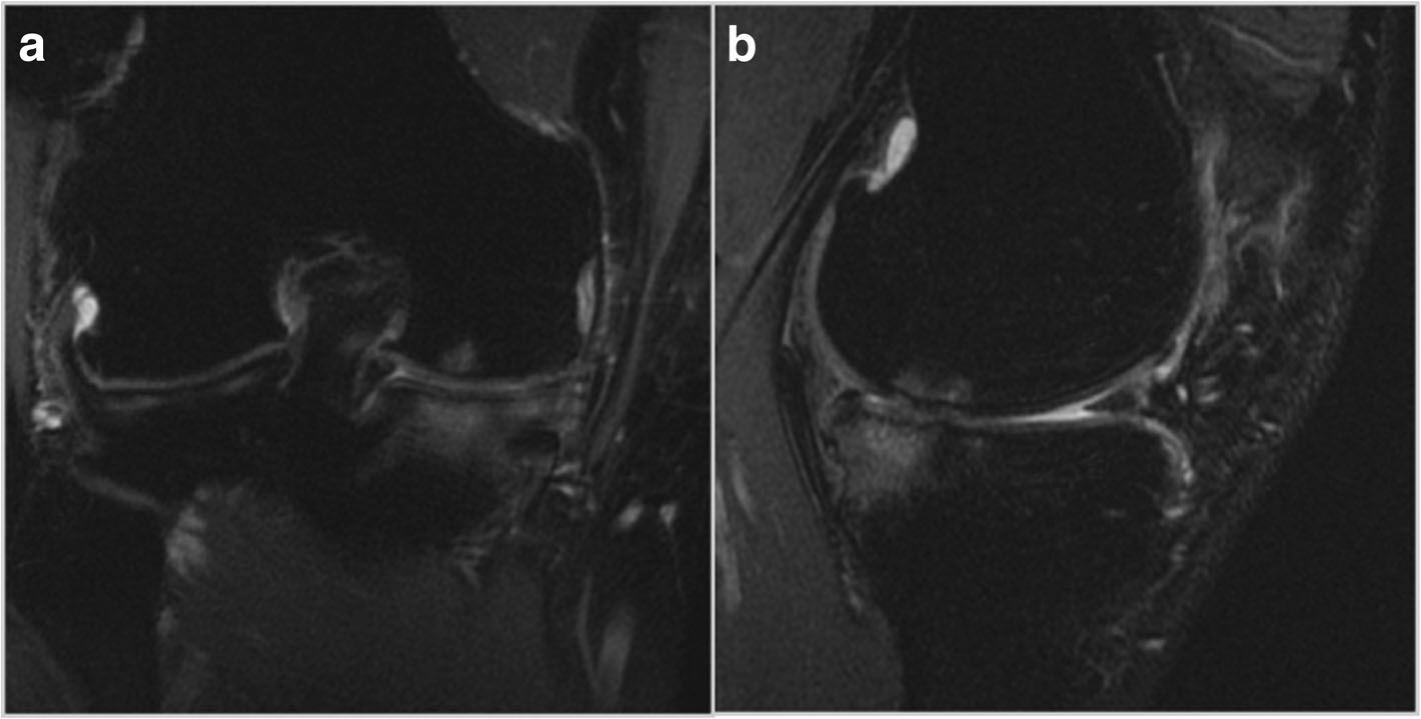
A recent frontal (a) and sagittal (b) MRI Patient 1
